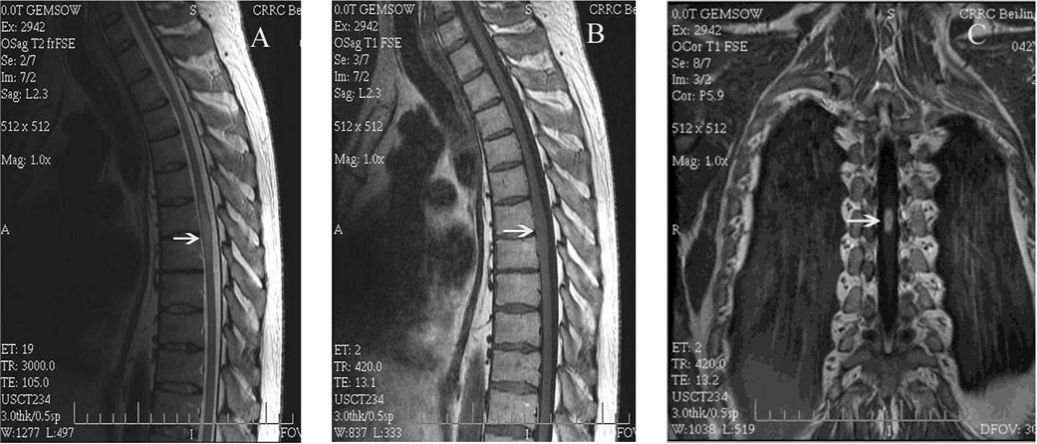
The second MRI images of the thoracic spine. A) Sagittal T_2_-weighted MRI image shows a hyperintense lesion expanding the cord at the T_6–7_ spinal level. B) Sagittal T_1_-weighted MRI image shows an isointense lesion expanding the cord at the T_6–7_ spinal level. C) Coronal T_1_-weighted MRI image shows a ring-like enhancing intramedullary lesion (16 × 7 × 6 mm) with gadolinium.
